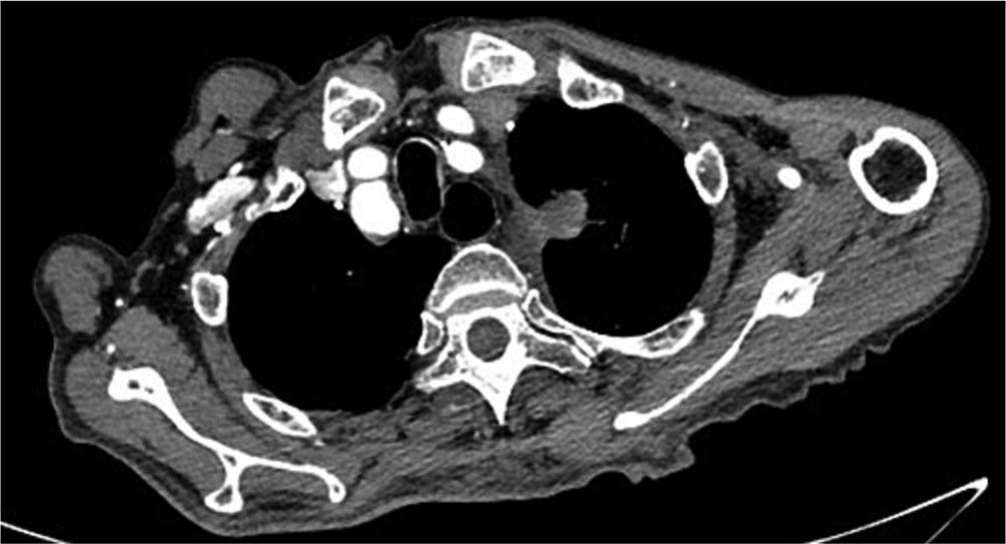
Lung CT results revealed no lung lesions associated with blood in sputum.
